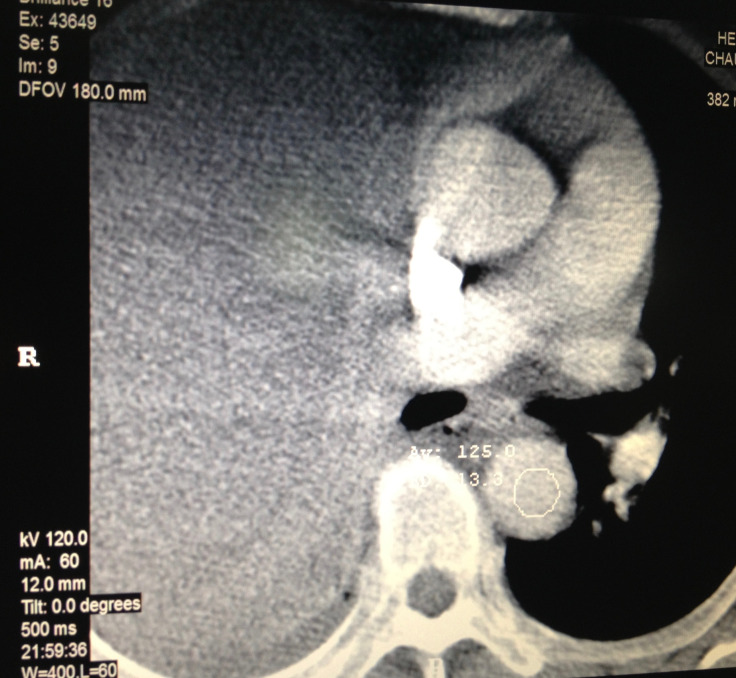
An axial computed tomography scan showing a homogeneously
